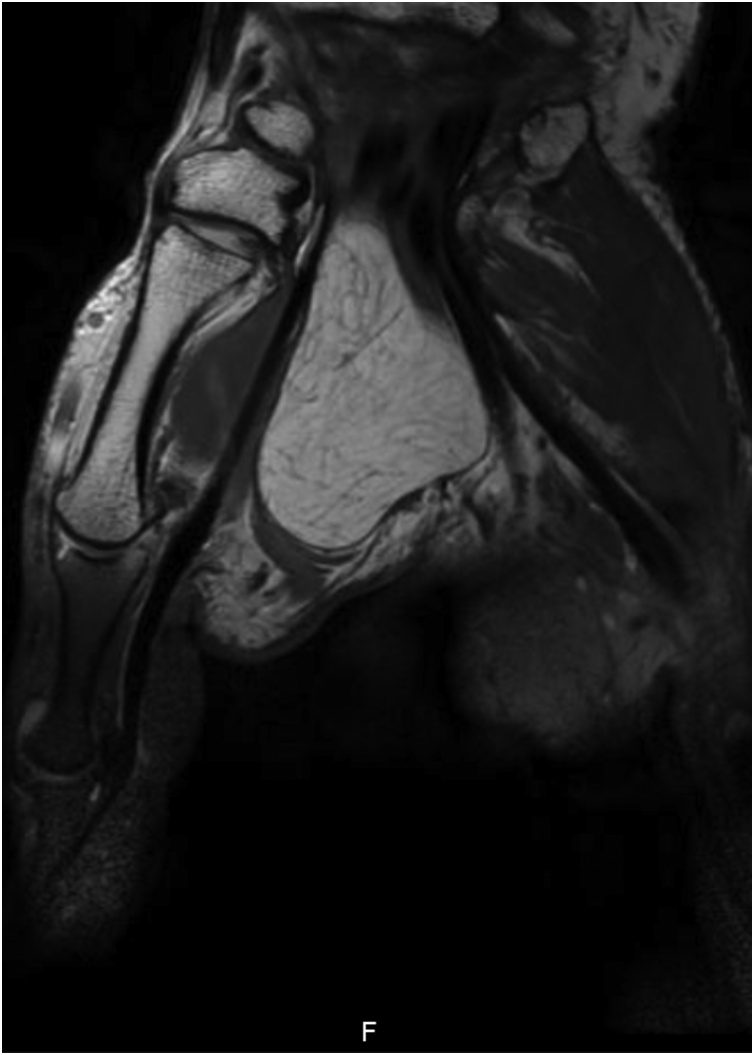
T1-weigthed coronal MRI image showing a capsulated adipose tumour without tendon involvement.
